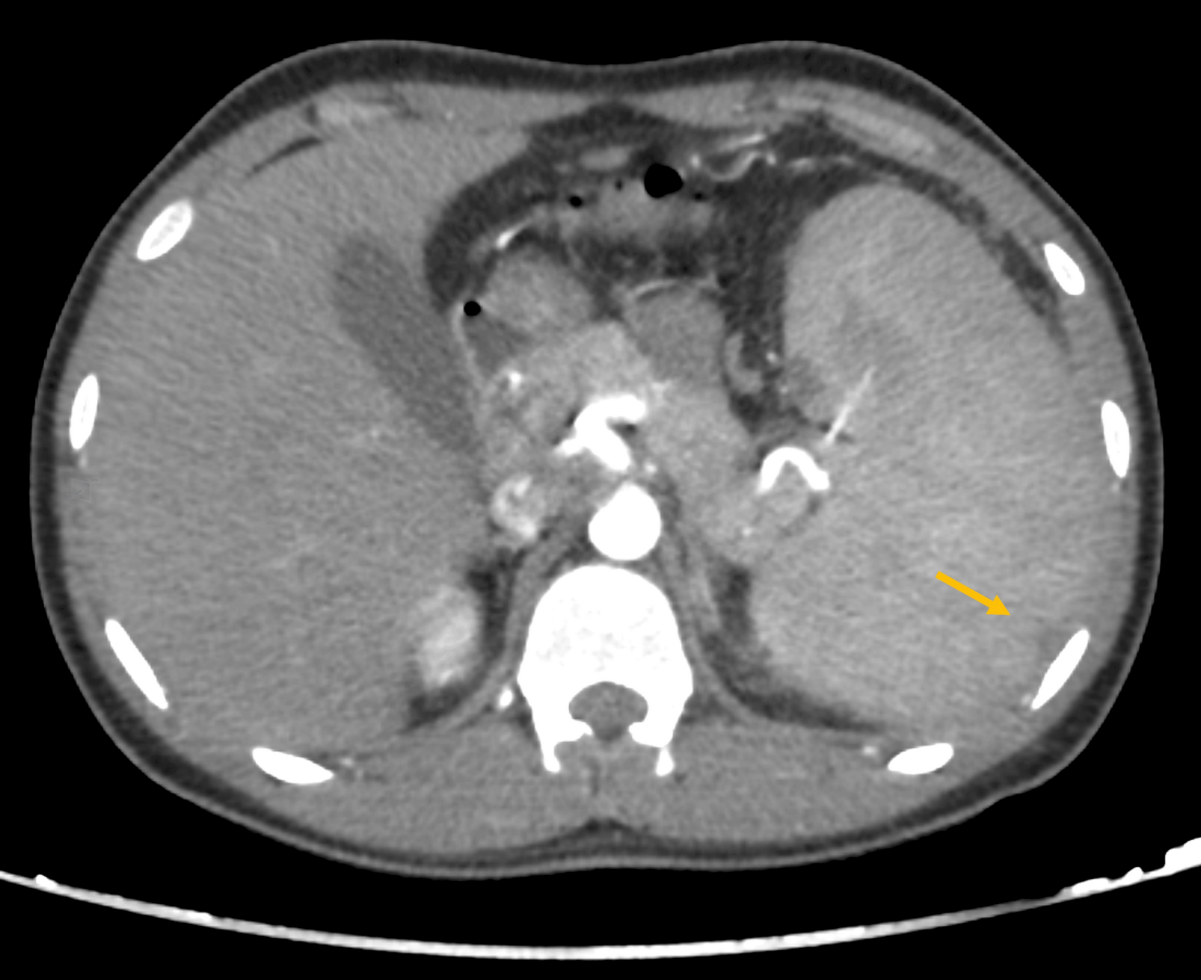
Abdominal CT scan (arterial phase) with an IV contrast axial cut demonstrating a mildly enlarged spleen with at least two small peripherally located splenic lacerations (arrow) measuring approximately up to 1 cm
